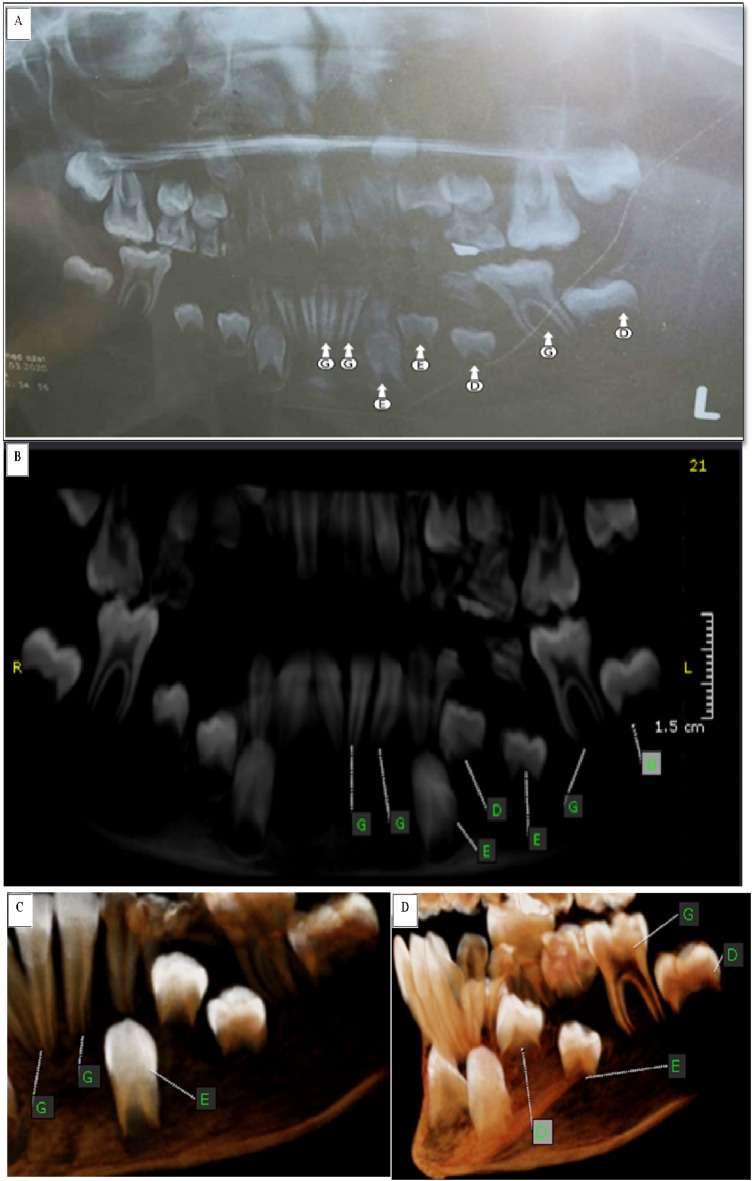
(A) panoramic radiograph of a 9-year-old acute lymphoblastic leukemic male child showing different stages of developing lower left seven permanent teeth except for the third molar, scored according to Willems method. (B) a reconstructed panoramic view and (C, D) 3-D (teeth view) of CBCT showing some changes in scoring for the same teeth.
